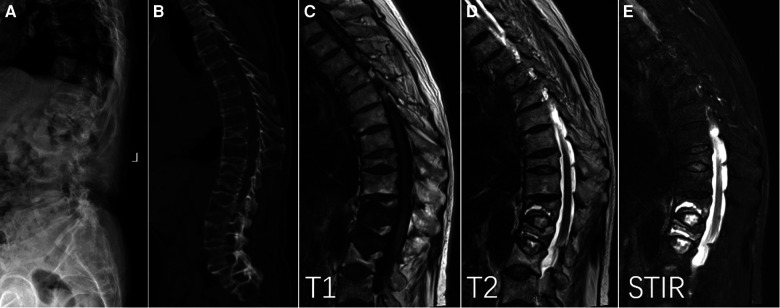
Intravertebral vacuum phenomenon (IVP) with vertebral fractures and ankylosing spondylitis. Lateral x-ray (A) and sagittal CT (B) showed low-intensity cleft throughout T12 and L1, characterized by well-defined hypointensity on T1 (C), hyperintensity on T2 (D), and hyperintensity on short time inversion recovery (E).
